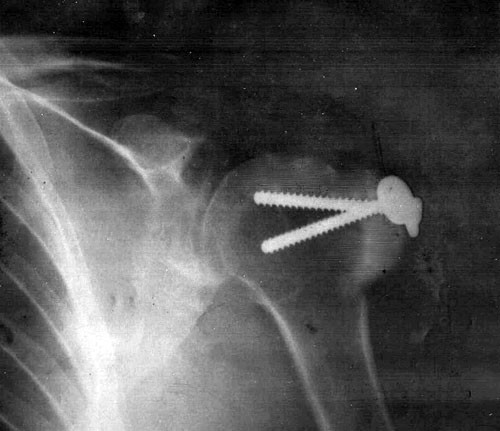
The radiograph shows that there is not downward subluxation of the humeral head.
